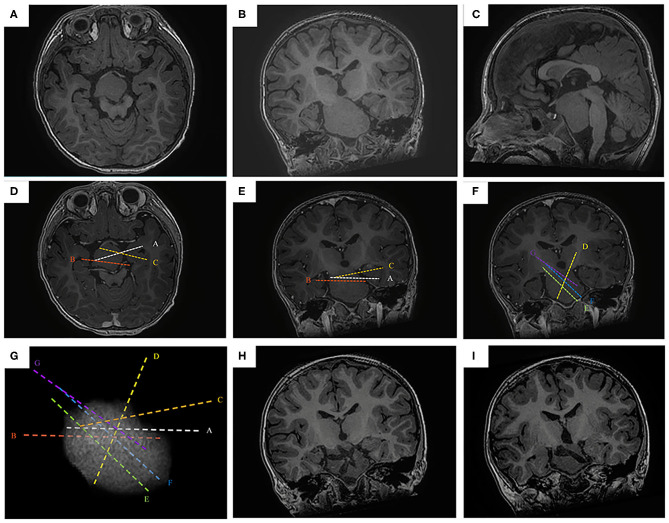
The SEEG-guided RF-TC in a giant HH with wide pedicel attached to hypothalamus. The preoperative MR images (A, axial image; B, coronal; C, sagittal) show a giant, wide pedical HH with left-sided dominant attachment to the hypothalamus (case No. 1). The electrodes are designed to cover the interface in the pedicel (D, axial image; E, coronal; F, sagittal). (G) the 3D demonstration of the electrode trajectory, the highest electrode density is at the base of HH. The postoperative MR images obtained 1 year after RF-TC (H,I, coronal image) shows the coagulated lesion at the base of the hamartoma. Note that the hamartoma was not coagulated totally, but focuses on the junction between the hamartoma and the hypothalamus.
